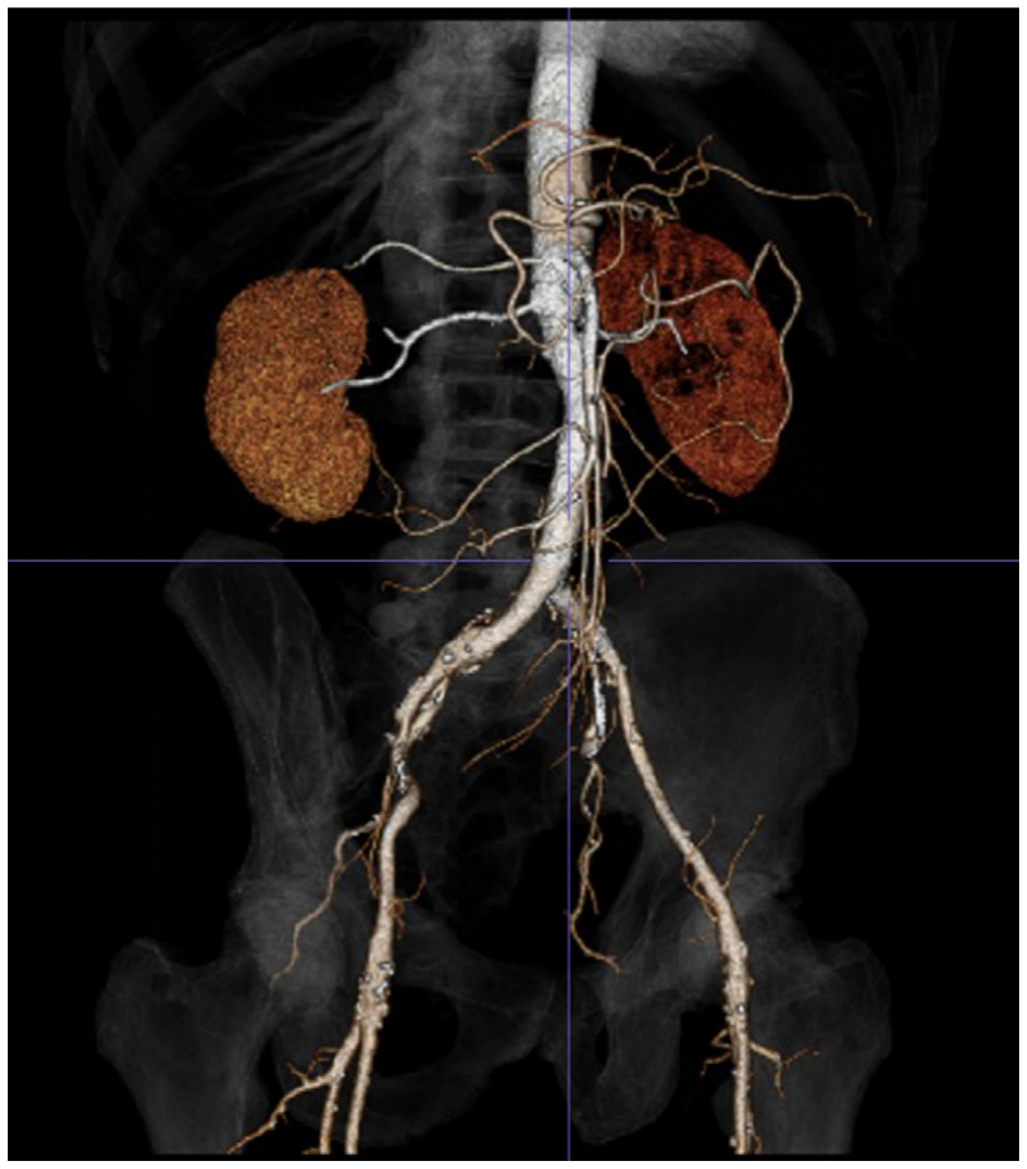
Postoperative computed tomography scan demonstrating patent stent graft at 33 months.
